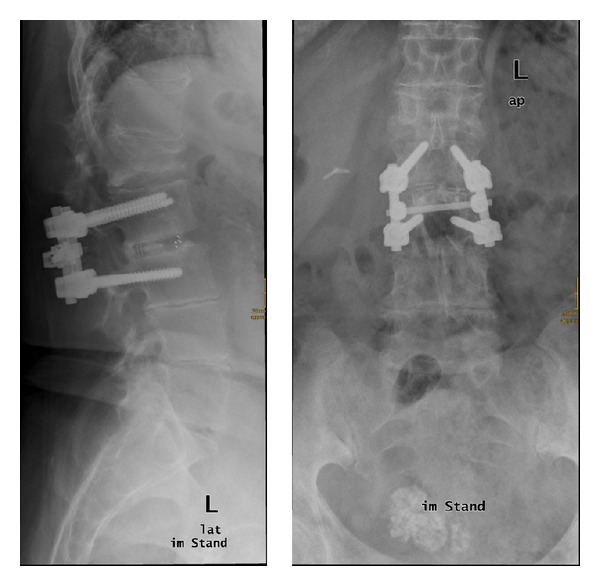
Preoperative X-ray in standing position in sagittal and antero-posterior plane and AP. Fresh fracture in L1. Prior operation was performed in L2/3 with a posterior lumbar intervertebral fusion (PLIF) 2 years ago.
